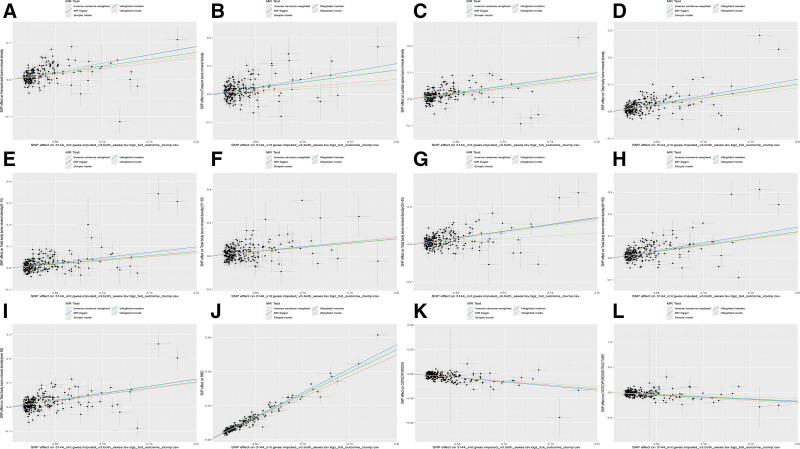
Scatter plots showing the causal relationship between heel broadband ultrasound attenuation, direct entry and the results obtained using different MR methods. Scatter plot of the causal relationship between heel broadband ultrasound attenuation, direct entry on (A) femoral neck BMD(B) forearm BMD, (C) lumbar spine BMD, (D) total body BMD, (E) total body BMD (0–15), (F) total body BMD (15–30), (G) total body BMD (30–45), (H) total body BMD (45–60), (I) total body BMD (over 60), (J) heel BMD, (K) osteoporosis, (L) osteoporosis fracture. The slope of each line corresponds to the causal estimate for each method. The background depicts the relationship between the effect of a single SNP on the outcome (points and vertical lines) and its effect on the exposure (points and horizontal lines). BMD = bone mineral density, MR = Mendelian randomization, SNP = single nucleotide polymorphism.
